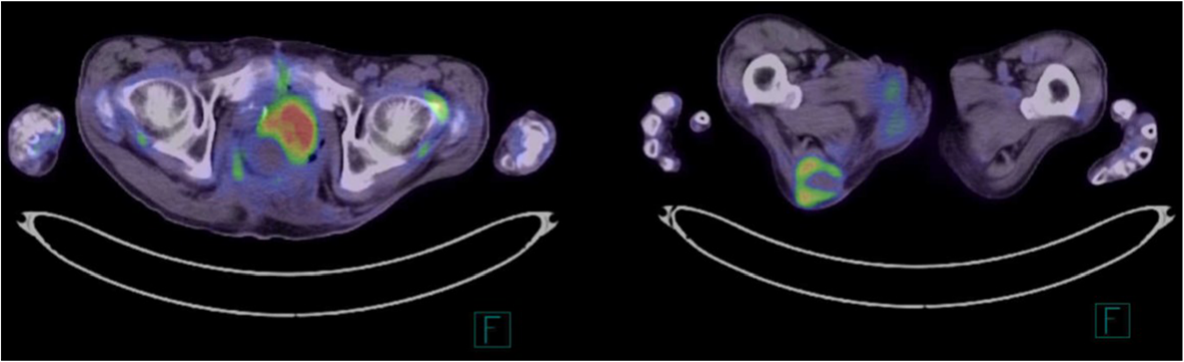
^18^F-fluorodeoxyglucose positron emission tomography scan demonstrated increased fluorodeoxyglucose uptake lesions in the perineal wound. The maximum standardized uptake values (SUV max) of the lesions were 6.36 and 13.45, respectively. Local recurrence was suspected because of an abnormal ^18^F-fluorodeoxyglucose positron emission tomography scan
